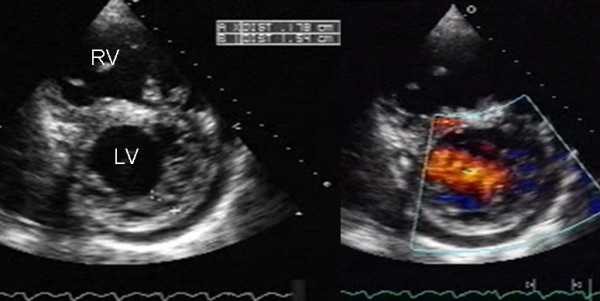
Two-dimensional parasternal and color Doppler images at the level of both ventricles that show the noncompacted:compacted wall ratio and how the color enters the intertrabecular recesses. Others abbreviations as before.
